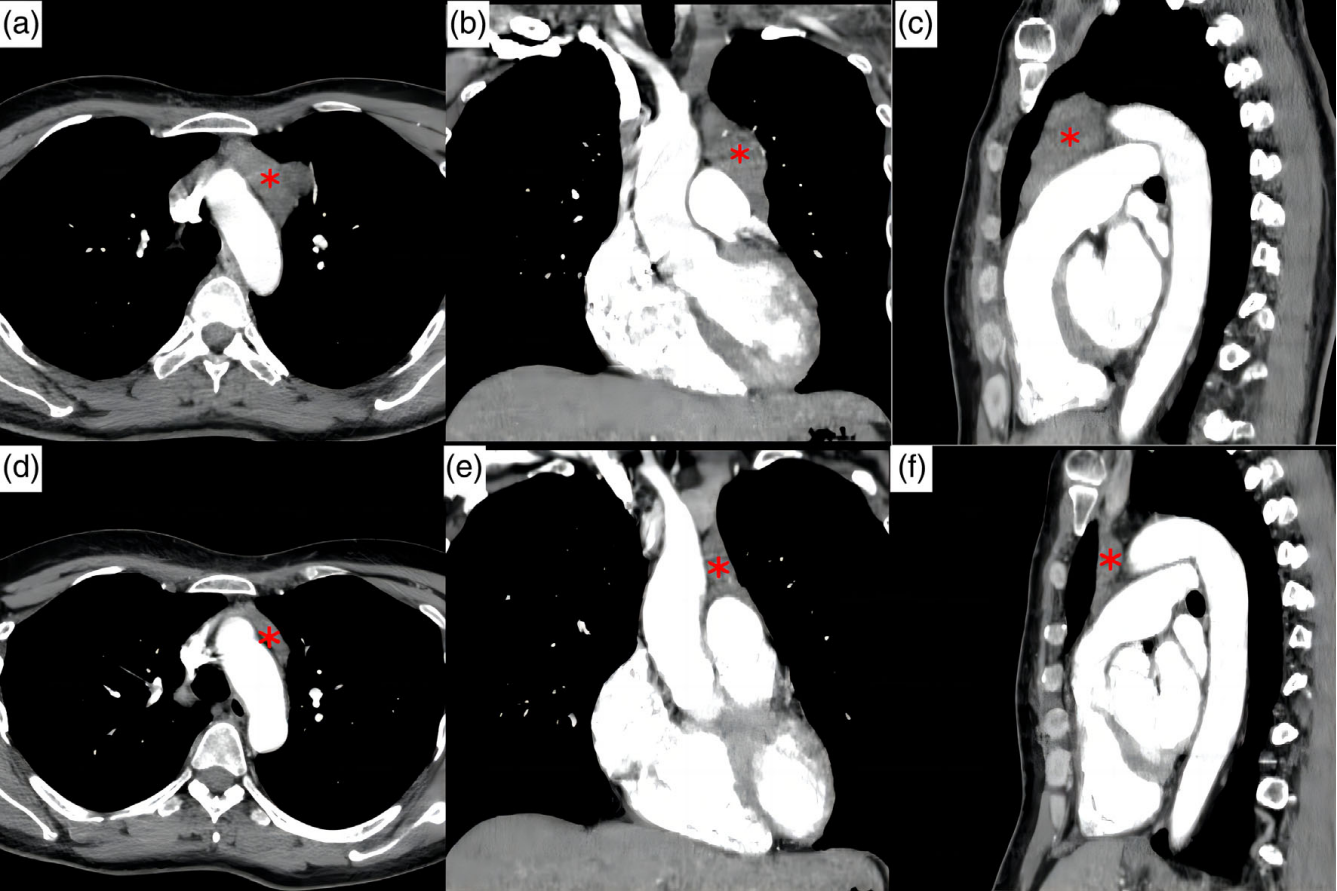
Chest enhanced computed tomography (CT) on admission. (a) Axial plane. (b) Coronal plane. (c) Sagittal plane. 127 mm × 42 mm (300 × 300 dpi). Repeat chest enhanced CT 4 months later. (d) Axial plane. (e) Coronal plane. (f) Sagittal plane. 127 mm × 42 mm (300 × 300 dpi).
